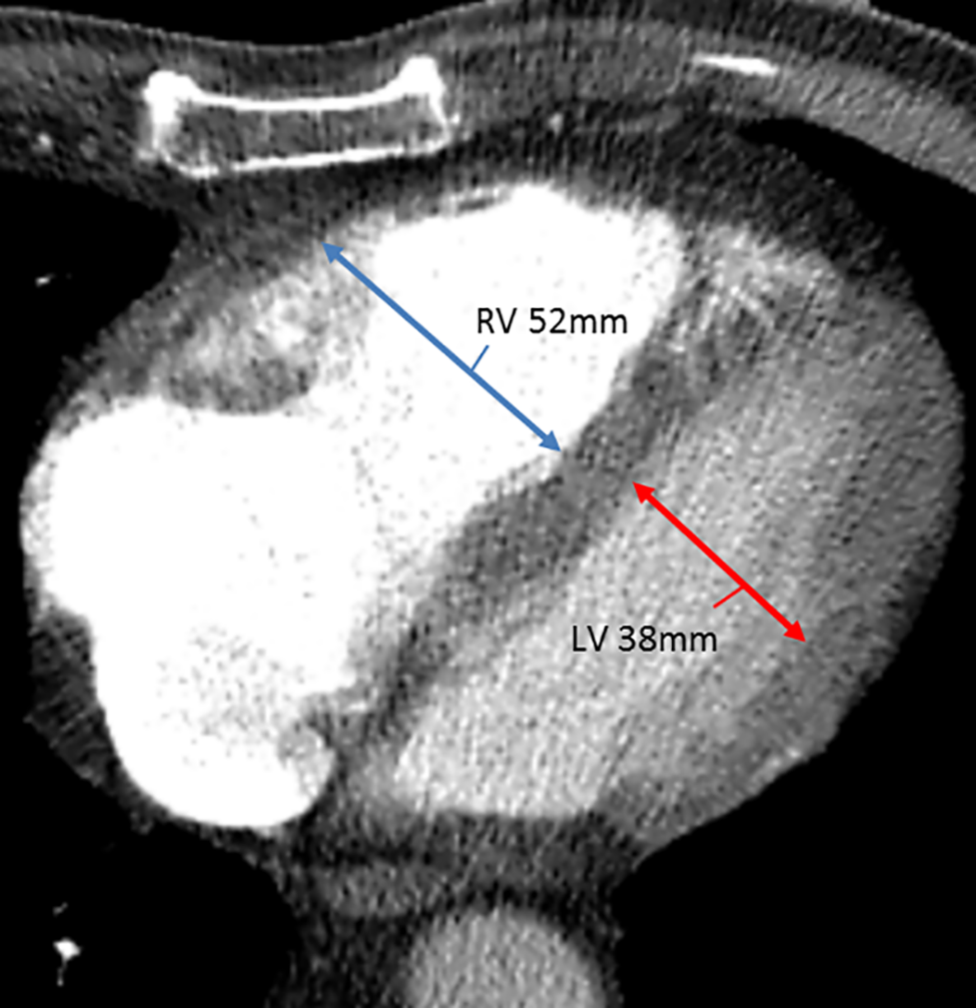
CTPA demonstrating the RV/LV ratio measurement. Note: CTPA: computed tomography pulmonary angiography; RV/LV: right-to left ventricle diameter ratio in this patient was 1.4.
